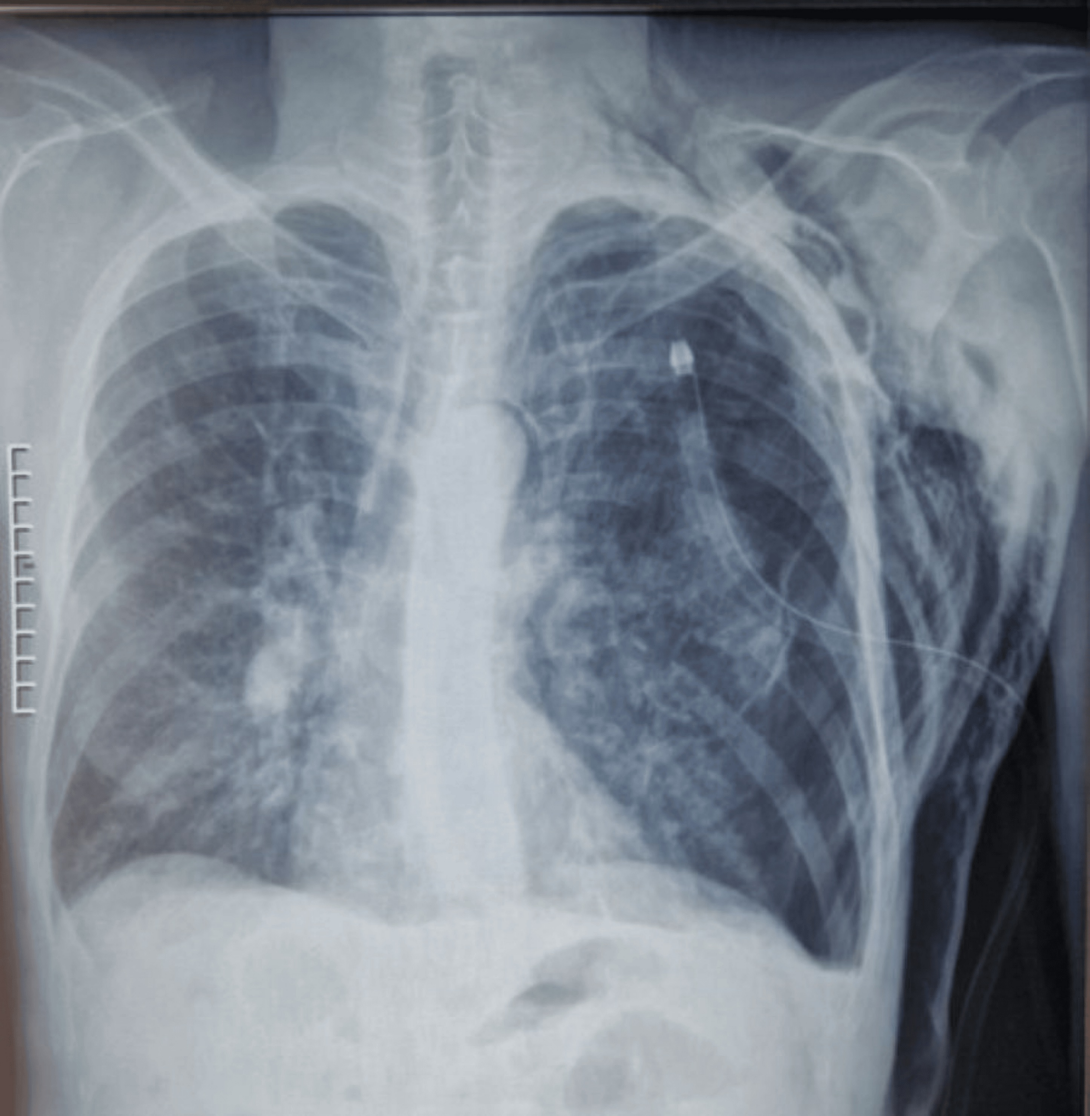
Chest X-ray taken one hour after chest tube insertion The chest X-ray shows a persistent pneumothorax associated with significant subcutaneous emphysema
